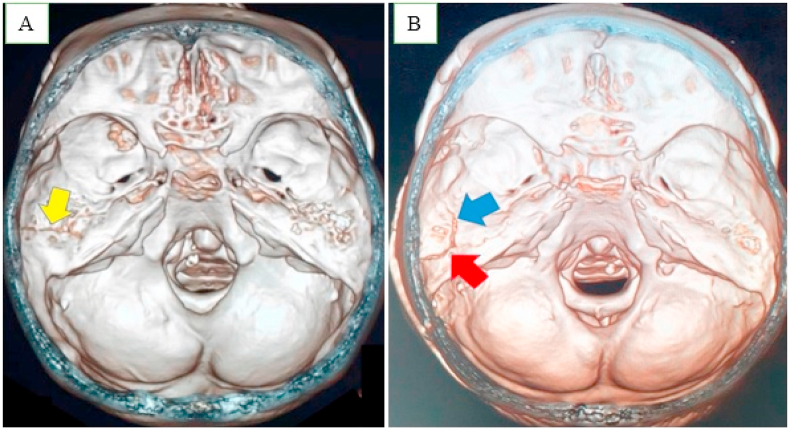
The 3D CT scan: A). a longitudinal fracture at first case (yellow arrow) and B). a longitudinal (red arrow) and transverse (blue arrow) fracture of petrous bone. (For interpretation of the references to colour in this figure legend, the reader is referred to the Web version of this article.)
